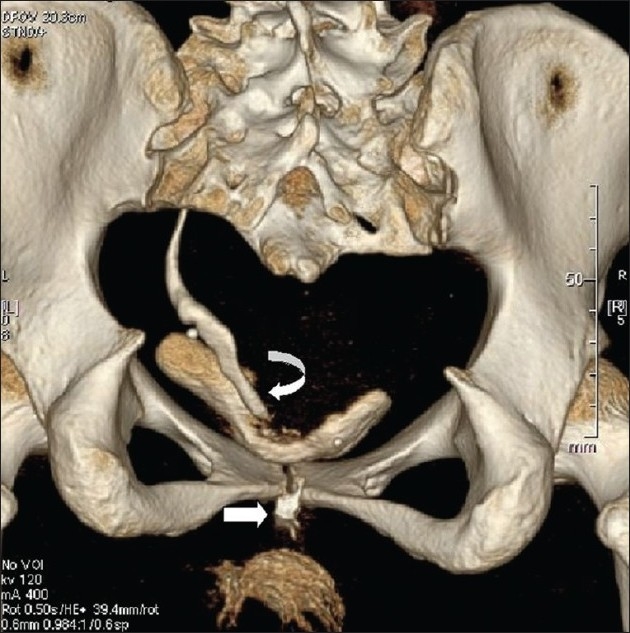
Three-dimensional reconstructed computed tomography image (posterior veiw); curved arrow showing insertion of the ureter and block arrow showing vaginal leak of contrast
